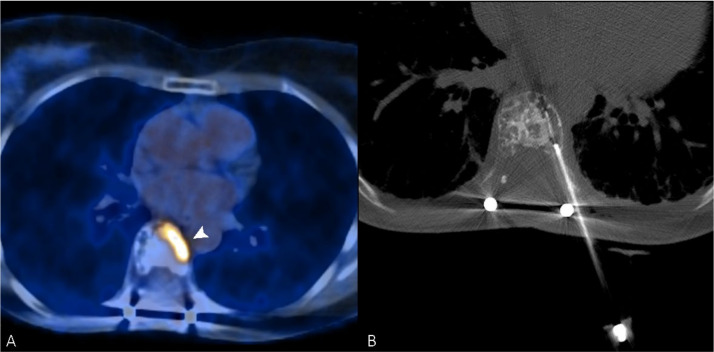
(A) PET/CT scan of a 56-year-old woman, which shows an intense uptake of 18F-FDG in correspondence with a painful vertebral metastasis from breast cancer in the body of T7 (arrowhead). (B) Radiofrequency ablation of the lesion performed through a transcostovertebral approach.
